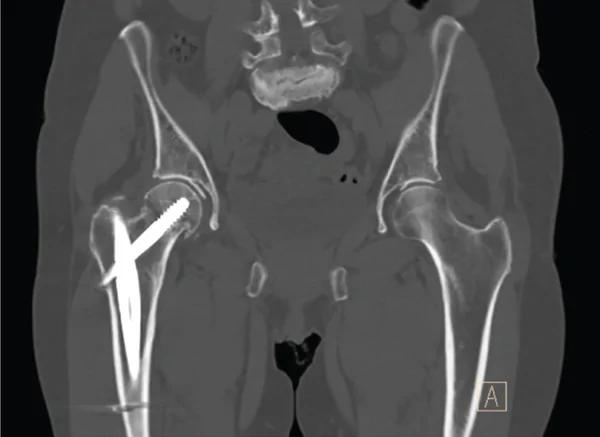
AP pelvis CT slice 9 days post subsequent injury.
